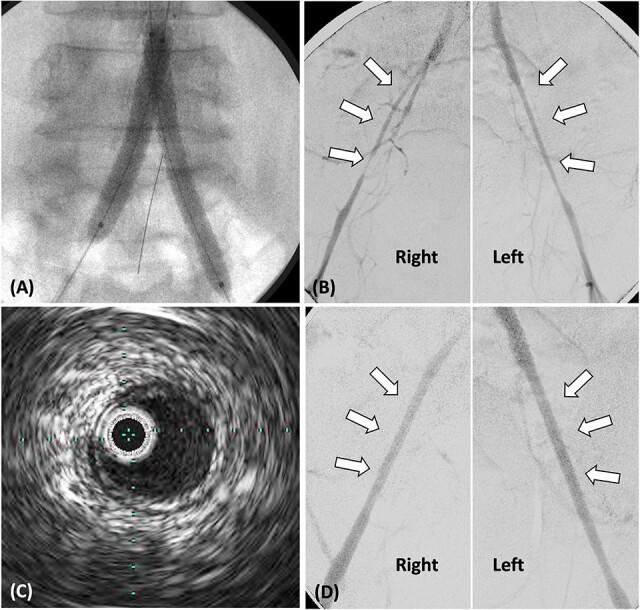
(A) Stent grafts placed from the abdominal aorta to bilateral CIA using the kissing stent technique. (B). Stenosis observed in both EIA, treated with plain balloon angioplasty only. (C) Post-balloon dilatation intravascular ultrasound reveals the absence of calcification or atheroma in both EIA. (D) Angiography post-balloon dilation reveals no EIA stenosis and calcification or atheroma.
